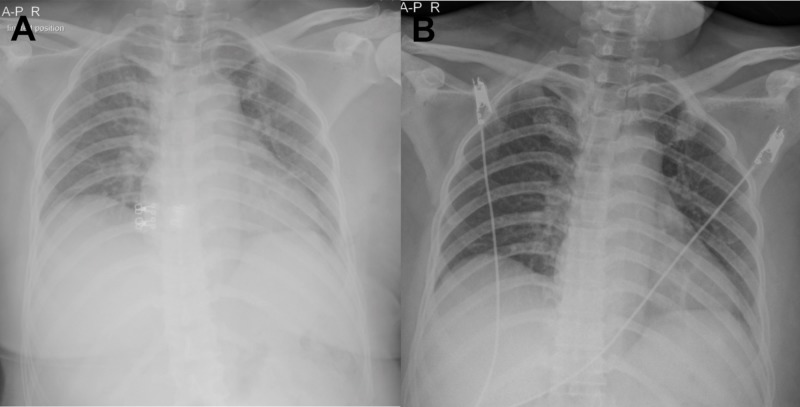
Chest X-rays During Treatment Course A) Chest x-ray showed bilateral hilar congestion six hours after aggressive fluid therapy during the plasma leakage stage of dengue hemorrhagic fever; B) Resolution of pulmonary congestion following the appropriate reduction of intravenous fluid.
